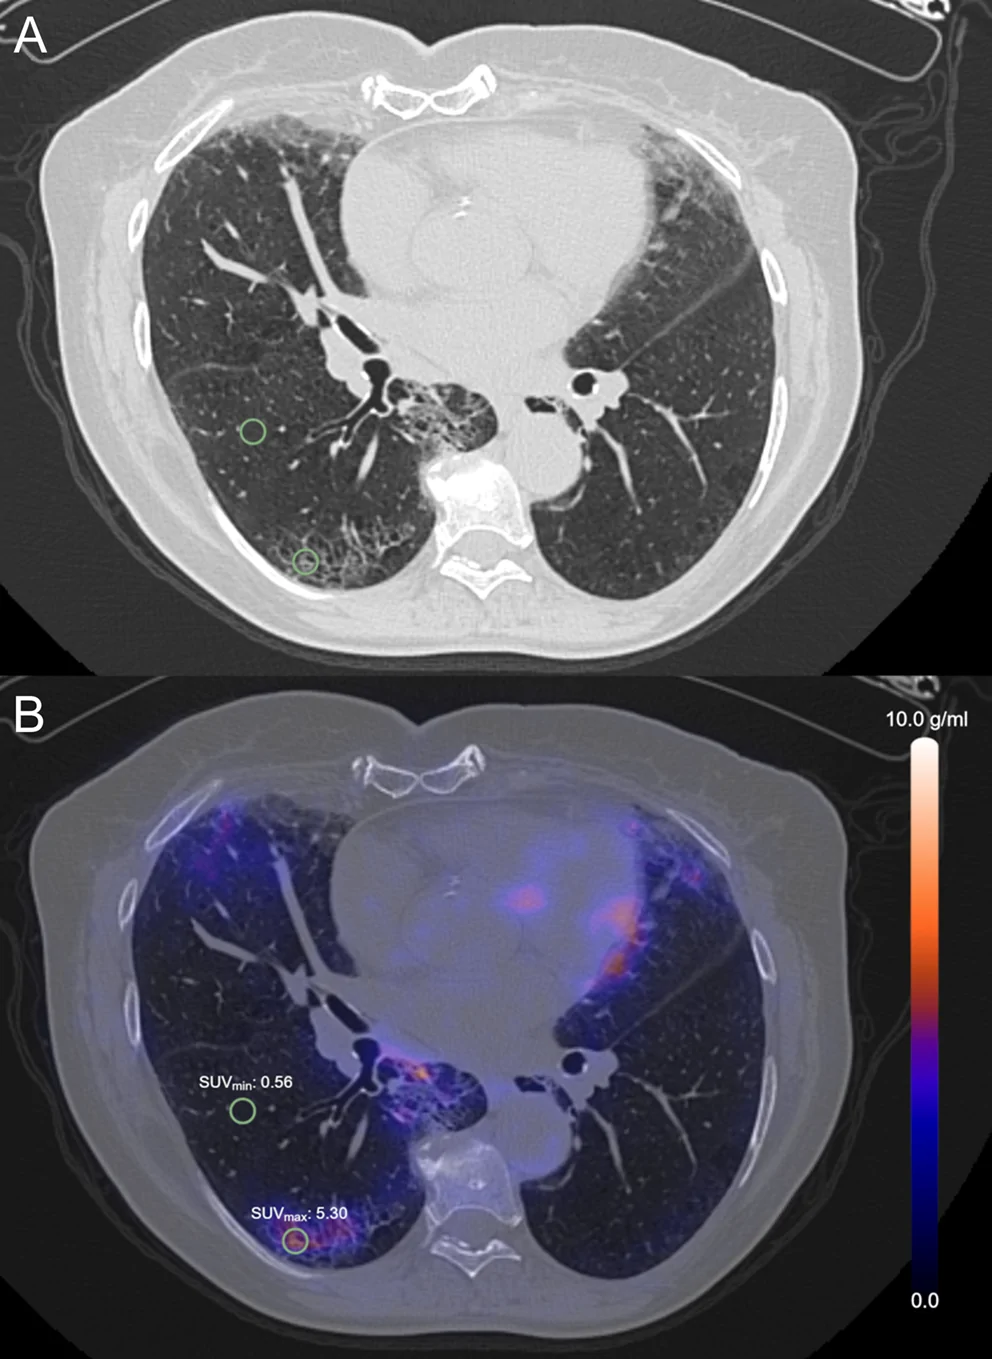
CT scan (A) and co-registered [^18^F]FDG PET/CT (B) of a patient with non-specific interstitial pneumonia, showing region of interest placement measuring maximal pulmonary [^18^F]FDG uptake (SUV_max_) and background uptake in unaffected lung (SUV_min_)
